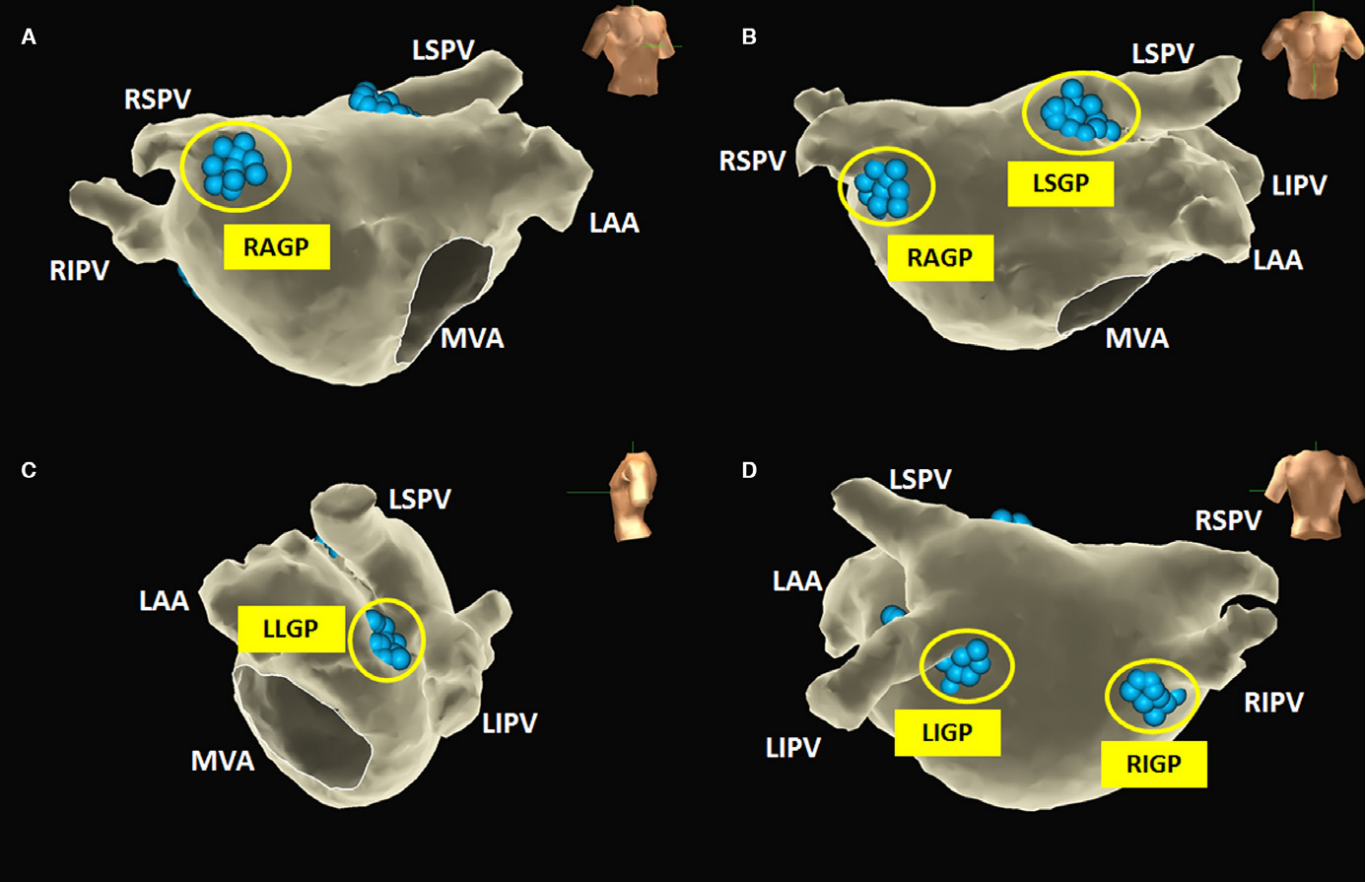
A 3‐dimensional computed tomography image of the left atrium showing the anatomical locations of the GPs. The blue balls represent the presumptive GP areas. A, The RAGP was well exposed in the right anterior oblique projection using electroanatomic guidance. B, The anteroposterior plus cranial projections helped identify the location of the LSGP. C, The LLGP between the LAA and LIPV was clearly exposed in the left lateral projection. D, The posteroanterior projection helped identify the locations of the LIGP and RIGP. GP indicates ganglionated plexus; LAA, left auricular appendage; LIGP, left inferior ganglionated plexus; LIPV, left inferior pulmonary vein; LLGP, left lateral ganglionated plexus; LSGP, left superior ganglionated plexus; LSPV, left superior pulmonary vein; MVA, mitral valve annulus; RAGP, right anterior ganglionated plexus; RIGP, right inferior ganglionated plexus; RIPV, right inferior pulmonary vein; RSPV, right superior pulmonary vein.
